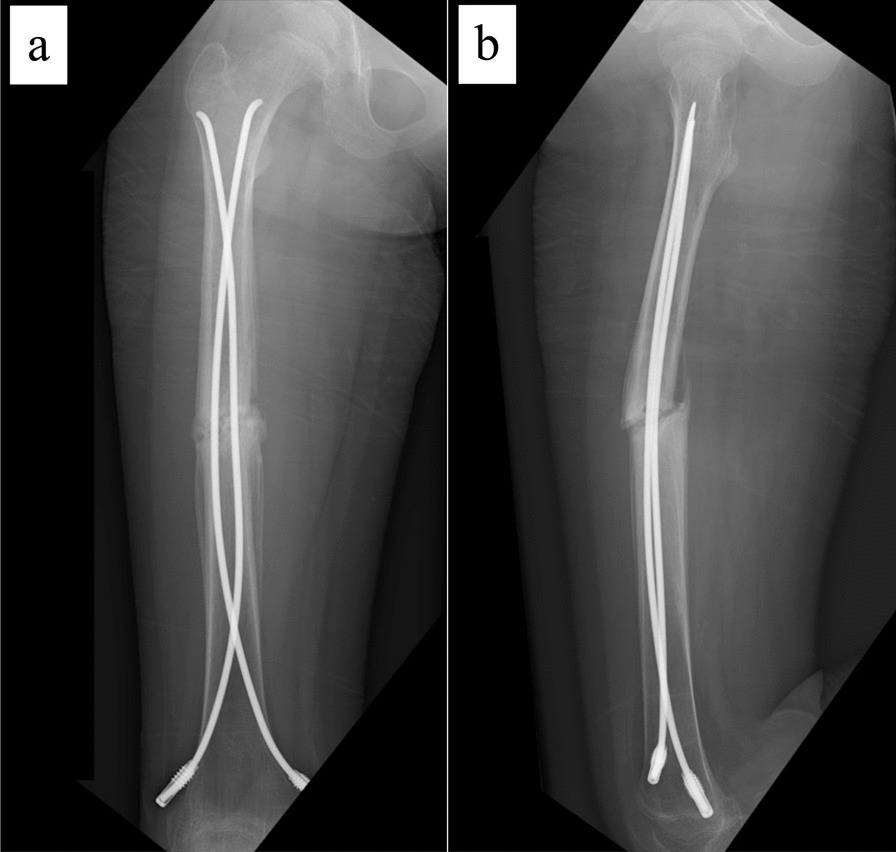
X-ray images at 6 months postoperatively. a Anteroposterior image and b lateral image. Nonunion is observed at the fracture site
